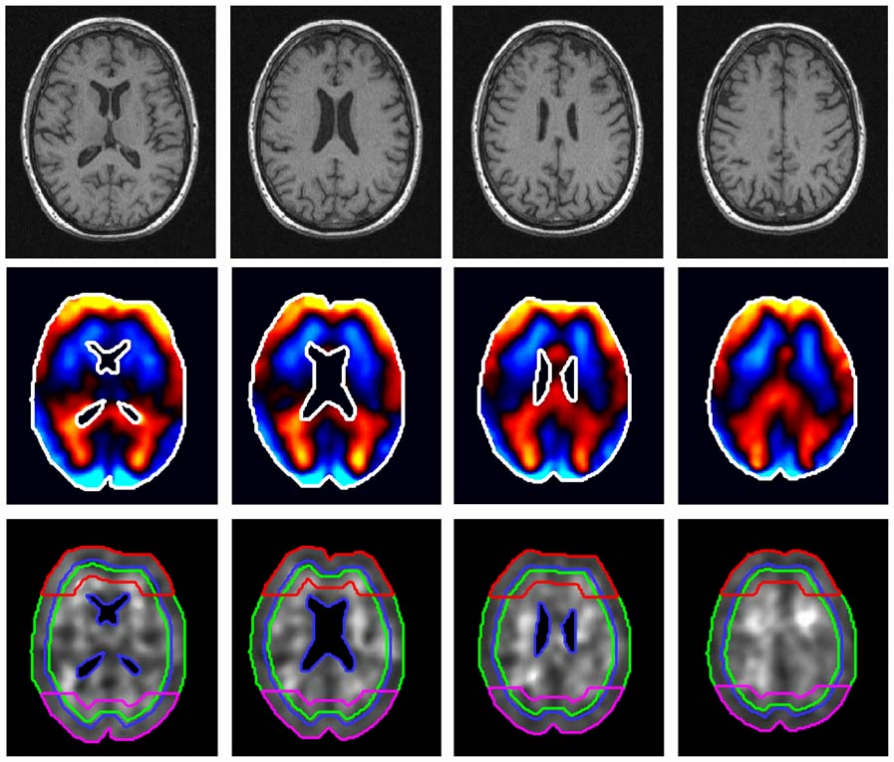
Four image slices from T1-weighted volume MRI data compliant with MRE slice positions (upper row). Color-coded MRE wave data of 50 Hz vibrations. Blue colors scale vibrations towards the reader, while red to yellow colors scale motion beneath the image plane. The maximum tissue deflection is approximately 80* µ*m (mid row). Real-part modulus images corresponding to 50 Hz vibration frequency with specific regions of interest (ROIs) investigated in this study. Green lines: *ROI*
